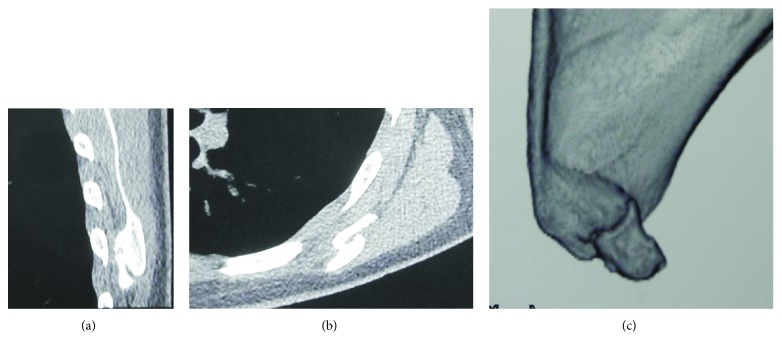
Computed tomography at the time of the first visit. (a) Sagittal section revealing that the callus did not connect the fragment with the scapular body. (b) Axial section demonstrating the lateral displacement of the fracture fragment. (c) Three-dimensional reconstruction image showing a bony protrusion ranging from the medial scapular border to the bony fragment, with a narrow break between the protrusion and fragment.
